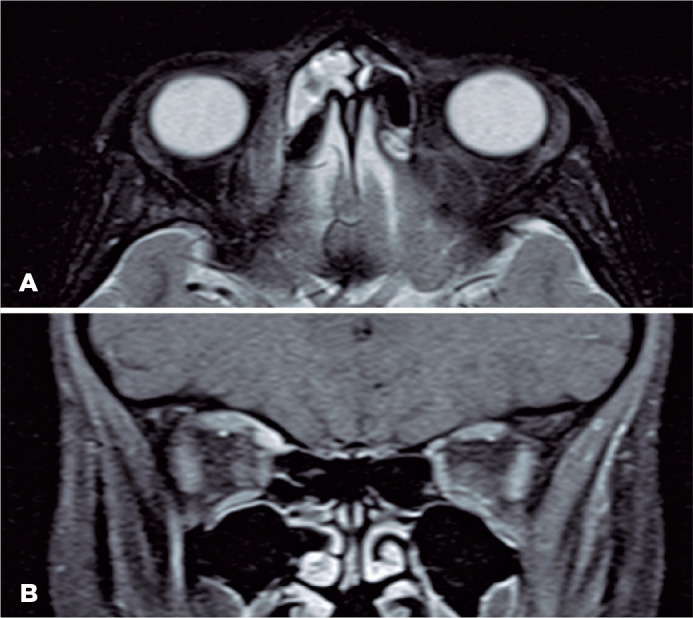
MRI of the orbits. A) Axial T2 without contrast and fusiform encut shows
